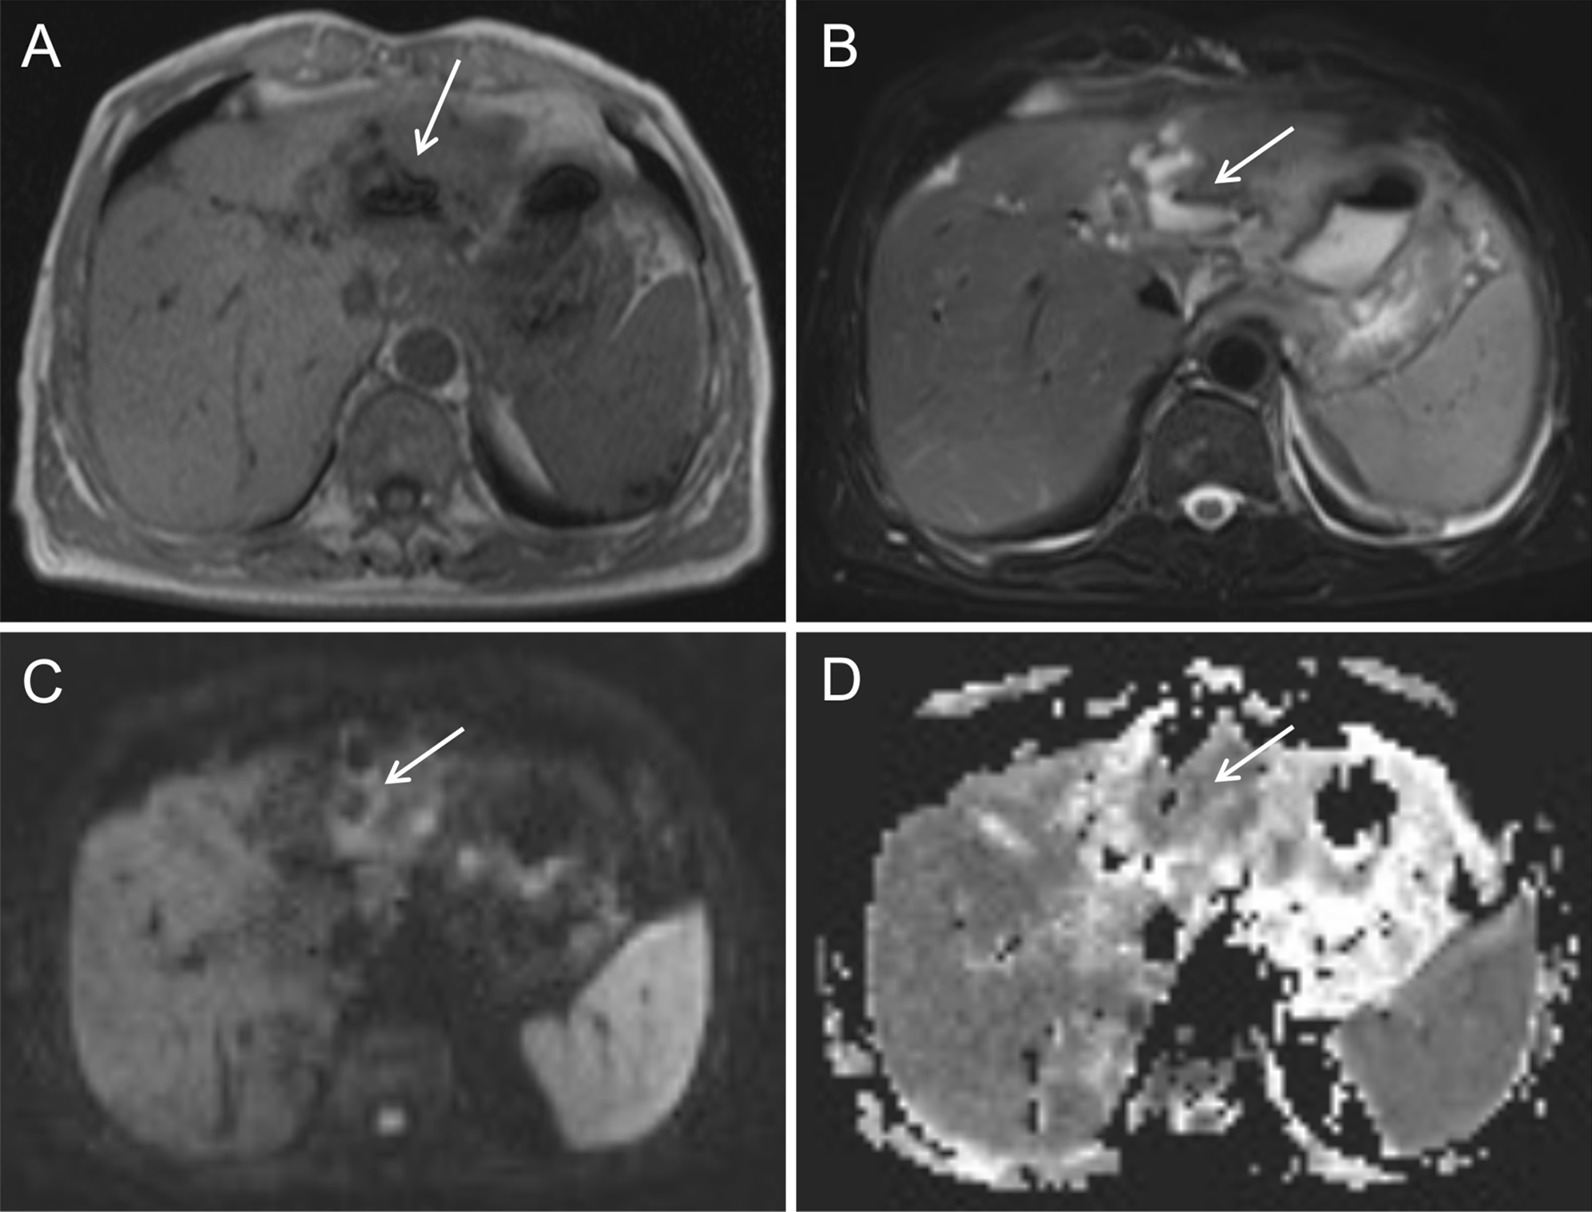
Plain MRI and diffusion-weighted images of case 3
